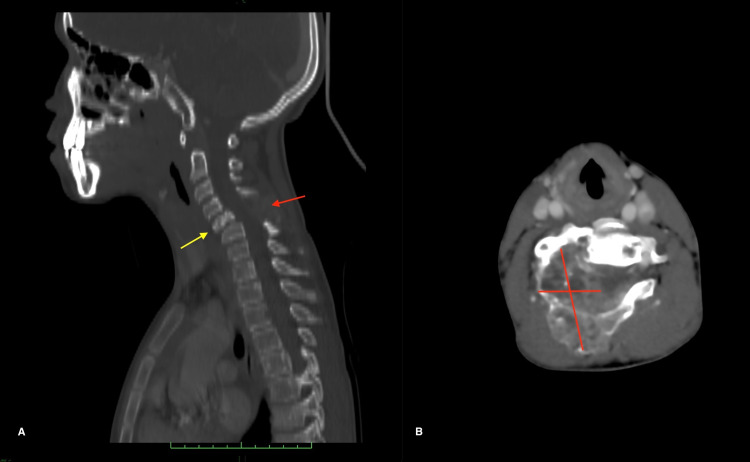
Sagittal (A) and axial injected CT scan (B) showing the heterogeneous lytic mass arising from C3 to C5 lamina (red arrow), compacting C5 body with cervical kyphosis (yellow arrow), encasing the right vertebral artery with preserved flow
